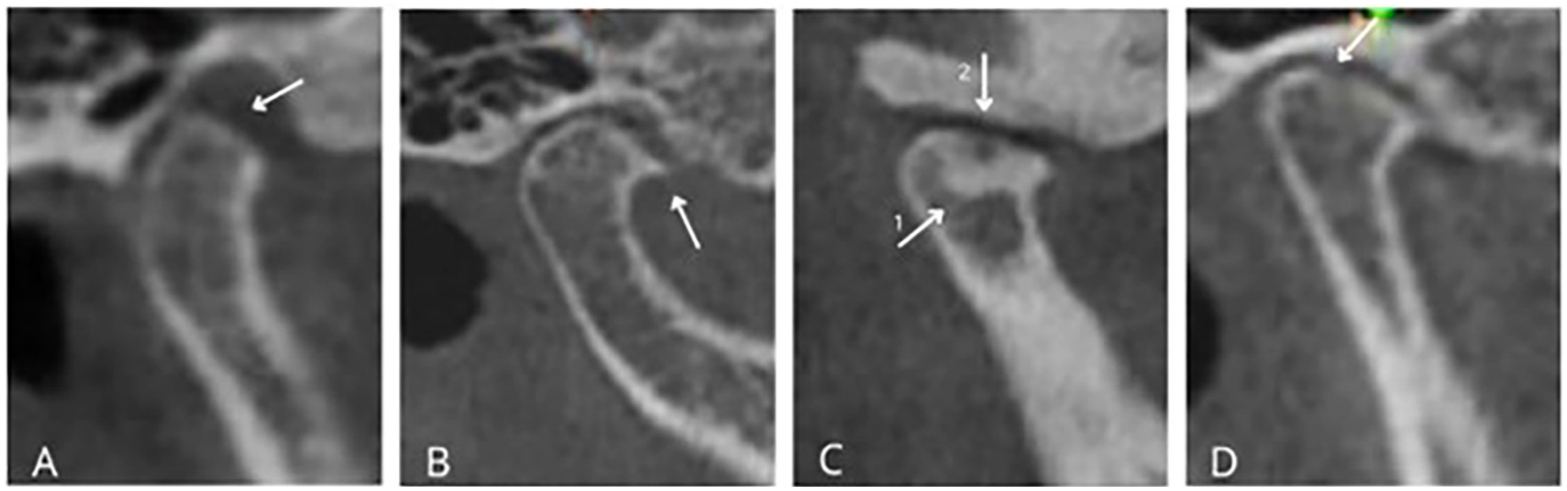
Radiographic signs of condylar osteoarthritis. A. Flattening; B. Osteophyte; C. (1) Sclerosis, (2) Cyst; D. Erosion.
